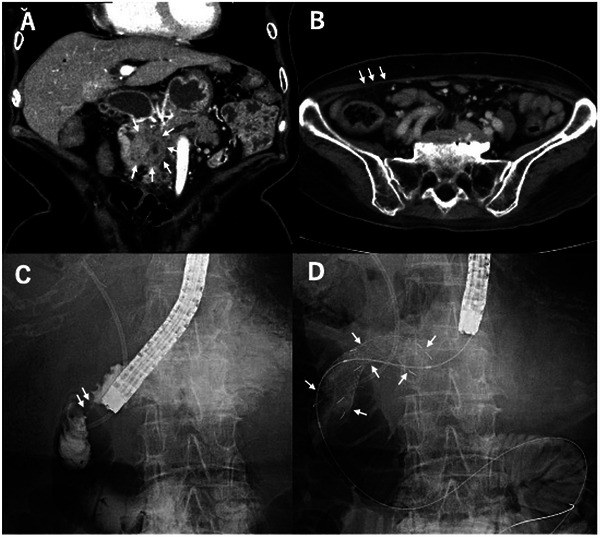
(A, B) Computed tomography (CT) image obtained before chemotherapy initiation. (A) Coronal CT image showing a 40‐mm mass in the head of the pancreas (white arrow) with upstream pancreatic duct dilatation and duodenal stenosis secondary to tumor invasion. (B) Axial CT image of the right lower abdomen showing no evidence of peritoneal dissemination, adhesions, or structural abnormalities at the site of subsequent stent impaction (white arrow). (C, D) Fluoroscopic images during placement of a duodenal stent. (C) Localized malignant stenosis at the duodenal bulb (white arrow). (D) Placement of a self‐expandable metallic stent (white arrow).
